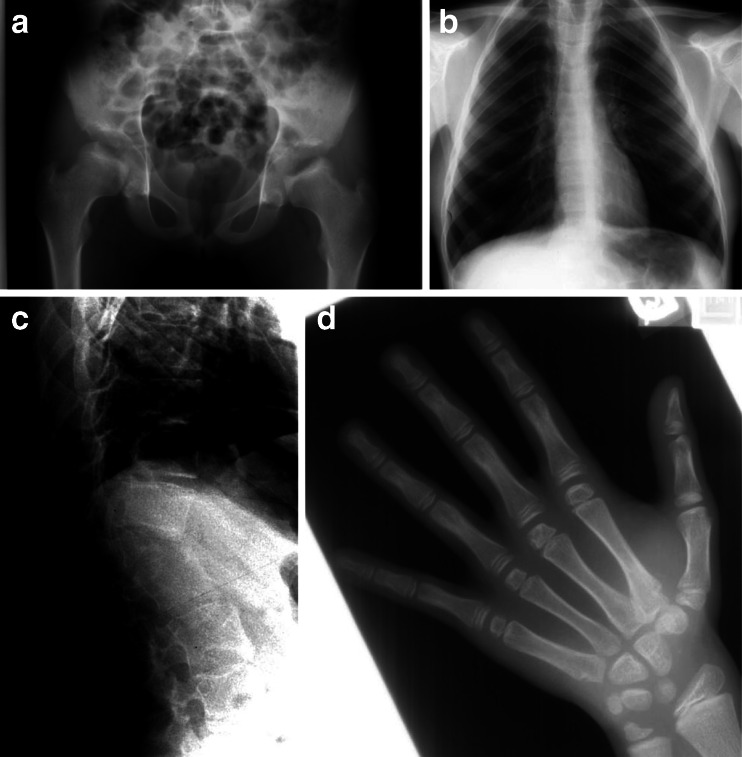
Radiographs of patient 1, female, Morquio A syndrome (MPS IVA). a Antero-posterior (AP) pelvis and hips, age 17 years: hypoplastic dense capital femoral epiphyses (CFE). b AP chest, age 17 years: normal. c Lateral spine, age 17 years: hypoplastic L1 vertebral body producing a significant gibbus. d AP hand and wrist, age 17 years: normal
